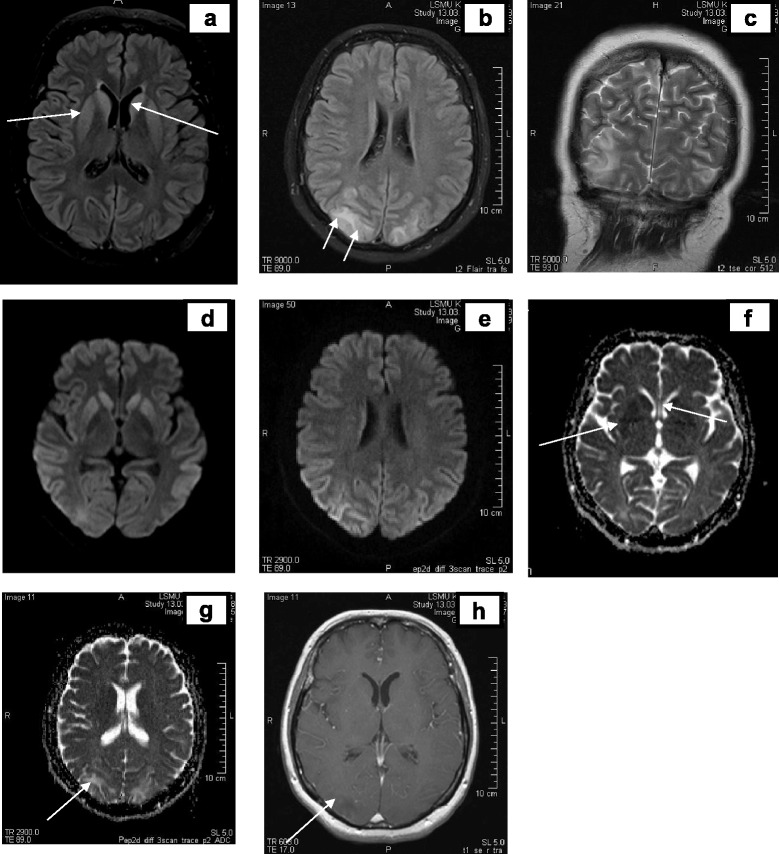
Magnetic resonance images at week 9. Legend: hyperintense lesions in T2W/FLAIR, T2W, DW, ADC (b, c, d, e, g) sequences in the occipital lobes cortex (*both sides*) and subcortical white matter (*in the right side*) *arrows* slight hiperintense lesions in bilateral subcortical nuclei (head of the *nucleus caudatus, n. lentiformis*) in T2W/FLAIR, DW (a, d, *arrows*) sequences with hypointensive signal SI lesions in ADC coefficient (f); hypointense lesion in the right occipital lobe in T1W sequence (h, *arrow*) with some contrast media enhancement dots
